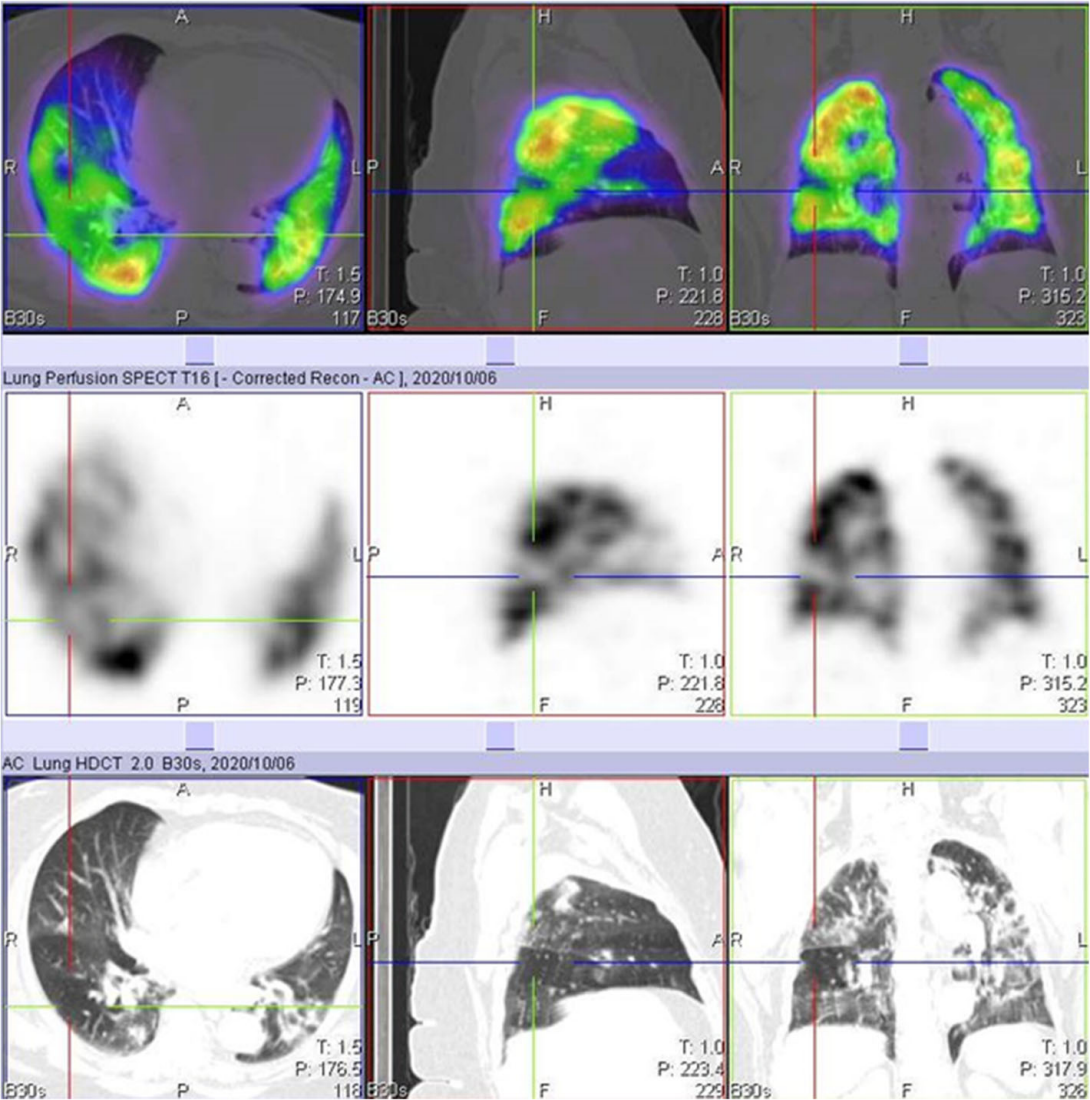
Perfusion only SPECT/CT images of a 42-year-old female, 13 days post COVID-19 infection, showing increased perfusion to areas of inflammation in the right lung, with a perfusion defect in an area without inflammation (crosshair) and associated mosaic hypoattenuation on CT
